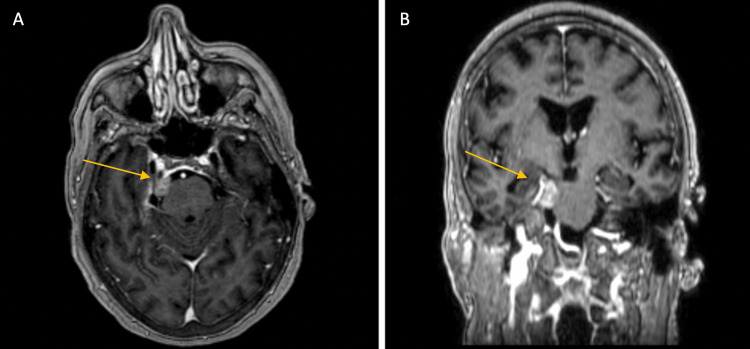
Contrast-enhanced MRI showing the right prepontine cistern lesion (A) axial and (B) coronal T1-weighted contrast-enhanced magnetic resonance imaging demonstrating an enhancing lesion in the right prepontine cistern along the tectorial incisura, superior to Meckel's cave, approximately 1.3 cm. This lesion abuts the cisternal segment of the right trigeminal nerve, which is deviated inferiorly.
